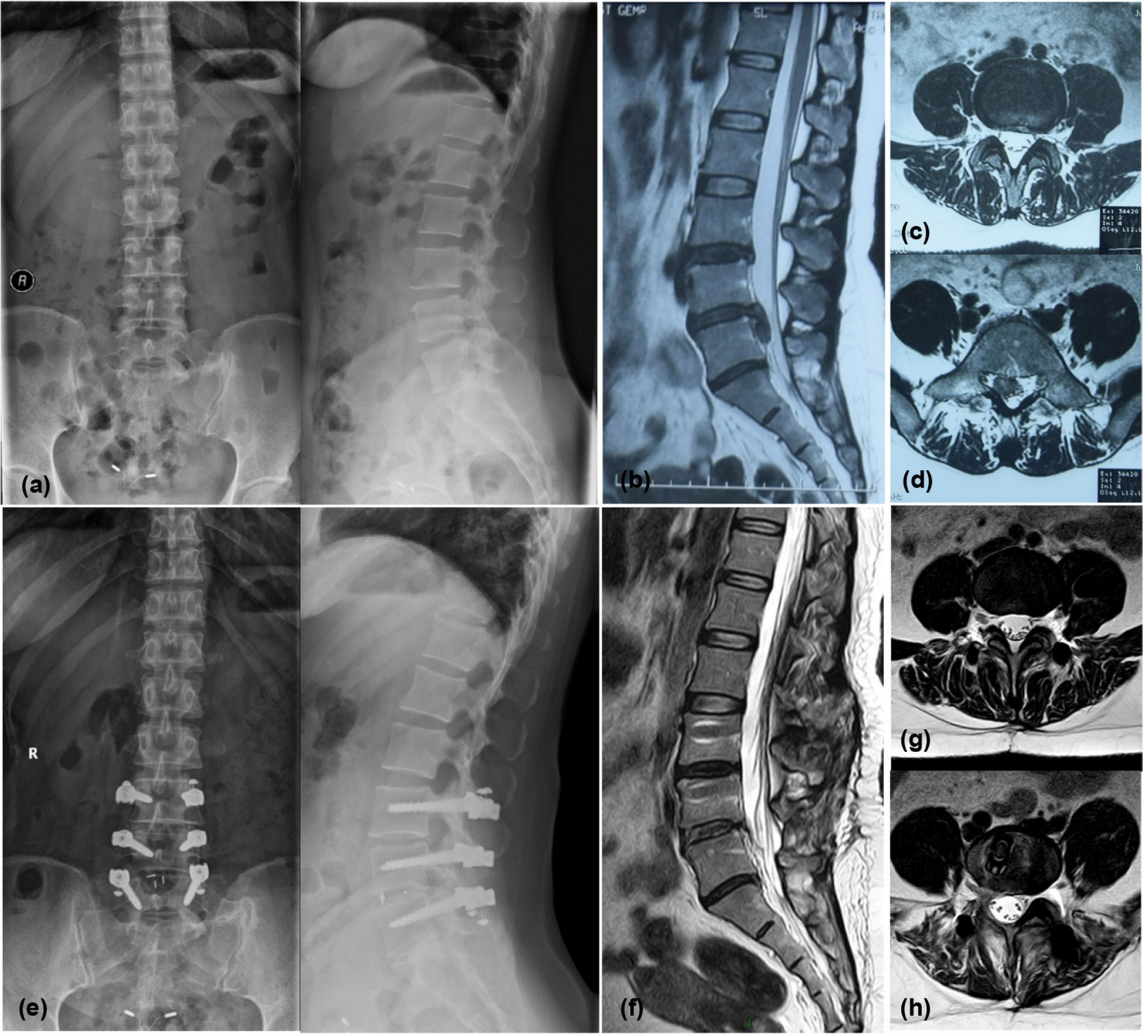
Typical case. The patient was a 40-year-old woman who was diagnosed with lumbar disc herniation (L4/5), cauda equina syndrome, and L3/4 disc degeneration and underwent L3-5 hybrid surgery with PEEK rods. a shows the lumbar X-ray before the operation. b shows the sagittal-plane MRI image of the lumbar region before the operation.c, d show the MRI images of the L3/4 and L4/5 sections of the lumbar region before the operation, respectively. e shows the lumbar X-ray at the 2-year follow-up. f shows the sagittal MRI image of the lumbar region at the 2-year follow-up. g, h show the MRI images of the L3/4 and L4/5 sections of the lumbar region at the 2-year follow-up, respectively. L3-5 were fixed segments, L3/4 was nonfused segments, the modified Pfirrmann classification of L3/4 was 6 both pre- and postoperatively, and the DHI was 0.28 preoperatively and 0.26 postoperatively. The modified Pfirrmann classification of the upper adjacent segment L2/3 was 2 both pre- and postoperatively, and the DHI was 0.33 preoperatively and 0.35 postoperatively. No significant degeneration was seen
